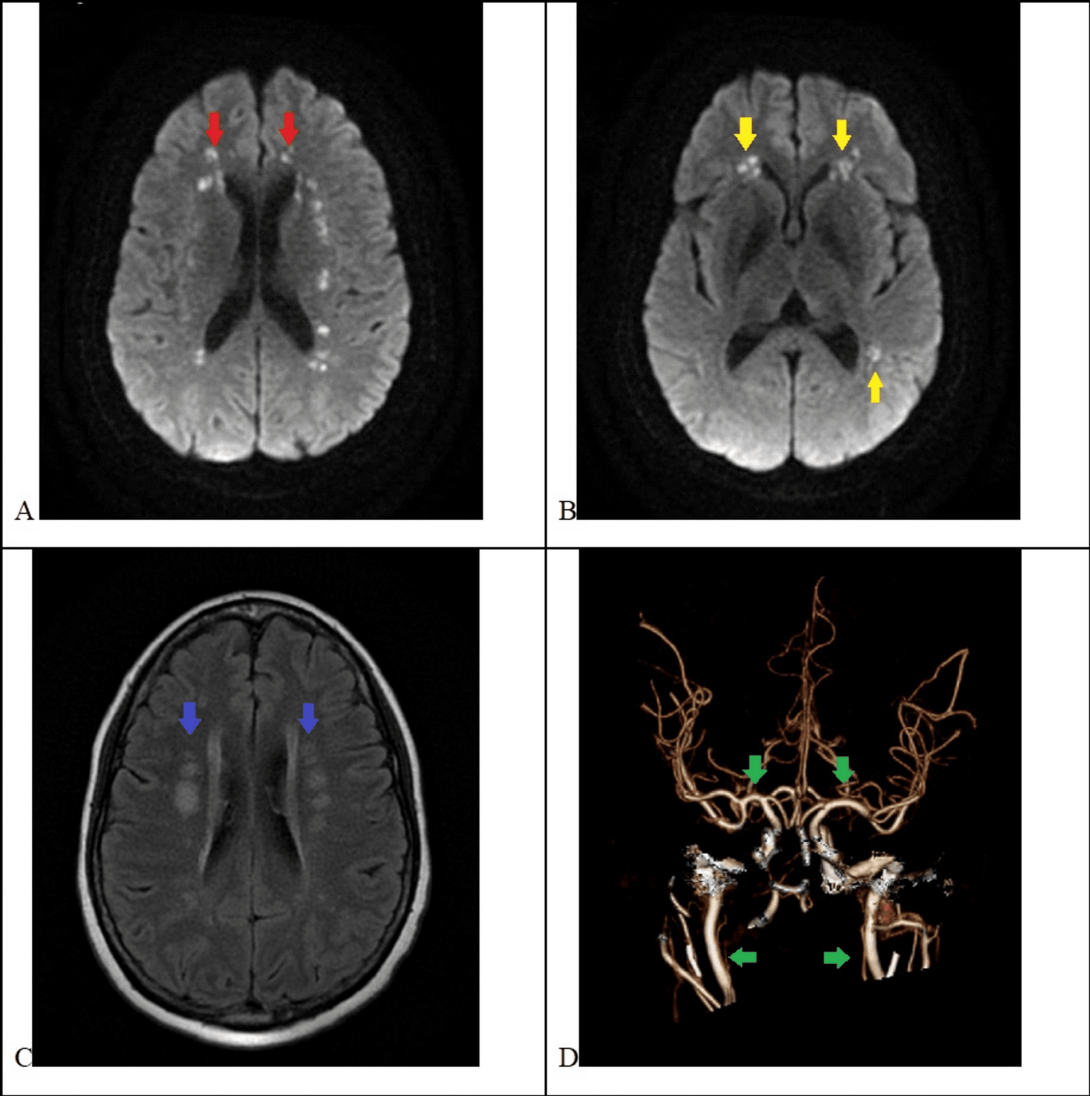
Brain magnetic resonance imaging with bilateral cortical and subcortical watershed strokes (A, B) MRI diffusion-weighted imaging (DWI) showing bilateral acute cortical (red arrows) and subcortical (yellow arrows) watershed strokes. (C) MRI T2 fluid-attenuated inversion recovery (FLAIR) showing bilateral subcortical watershed strokes (blue arrows). (D) CT angiogram of the head showing the circle of Willis with no high-grade stenosis in the anterior circulation (green arrows).
